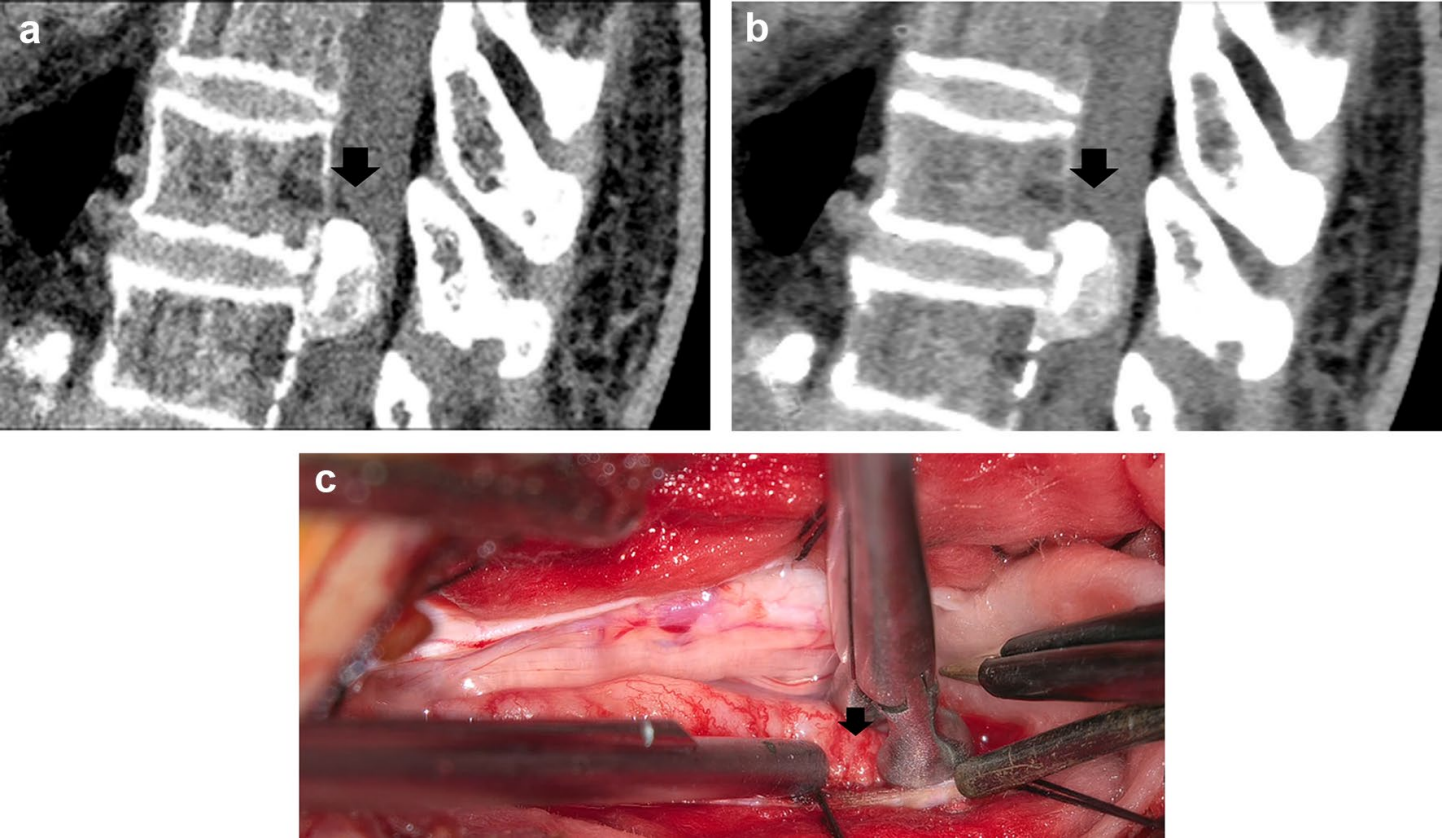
Spinal meningioma. In this case of a female patient in her 50 s with numbness in the plantar region, MRI T1WI showed an iso-intensity mass and T2WI showed a low-intensity mass at the Th10–11 level in the spinal canal. Gd-T1WI sagittal images showed strong homogeneous enhancement and a dural tail sign. PCD-CTA (kernel Qr60 and Qr44) revealed a 21-mm mass with homogeneous enhancement on the right side of the spinal canal, a dural tail sign, and coarse calcification. In contrast to Qr60 and Qr44, the coarse calcification of Qr60 (a) is more clearly delineated than that of Qr44 (b). Intraoperative findings show granular calcification mixed with the tumoral tissues (c). The tumor was elastic and hard. *MRI* magnetic resonance imaging, *T1WI* T1-weighted imaging, *T2WI* T2-weighted imaging, *PCD-CTA* photon-counting detector-computed tomography angiography
